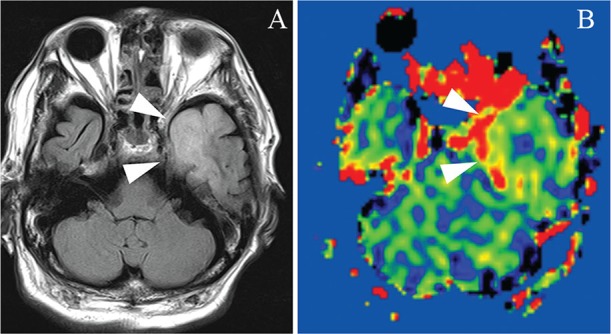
A 78-year-old man with non-purulent parenchymal involvement due to HSV infection. Fluid-attenuated inversion recovery shows a high intensity area in the left medial temporal lobe, which is typical in HSV encephalitis (A: arrowheads). The high perfusion in that area is pronounced on arterial spin-labeling magnetic resonance imaging (B: arrowheads). HSV, herpes simplex virus.
